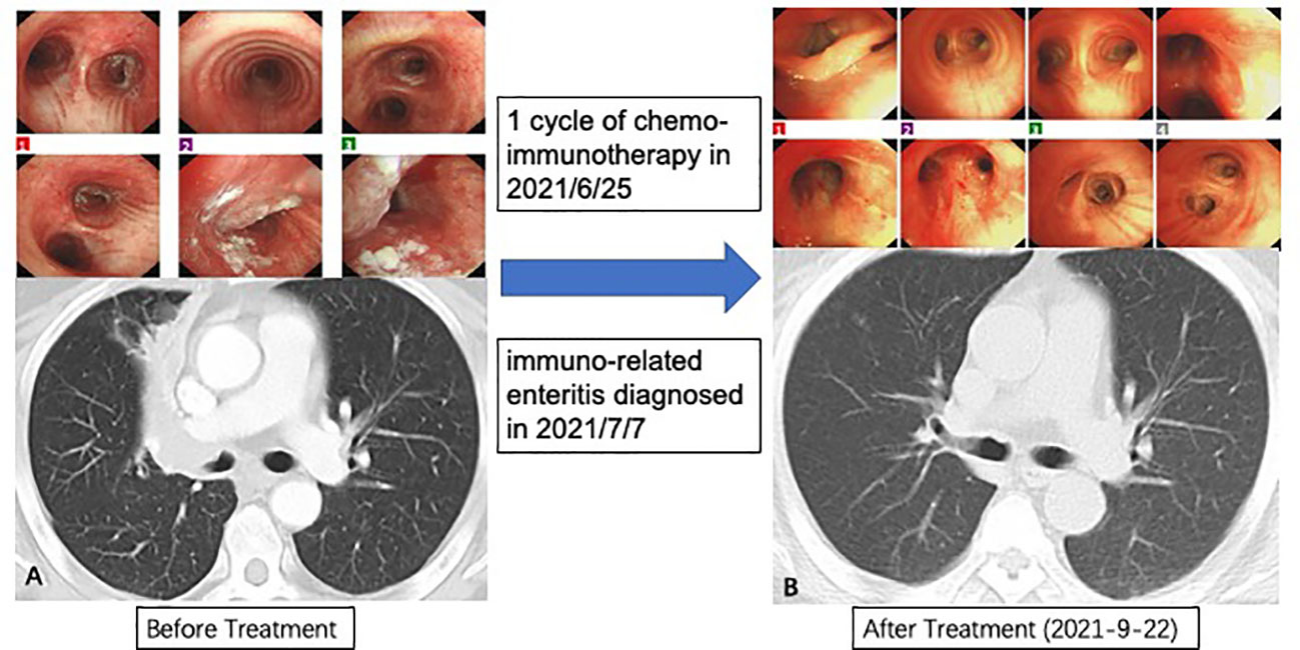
Procedures of treatment and response. (A) Baseline characteristic of lungs; (B) Great clinical response in bronchoscopy and computed tomography.
